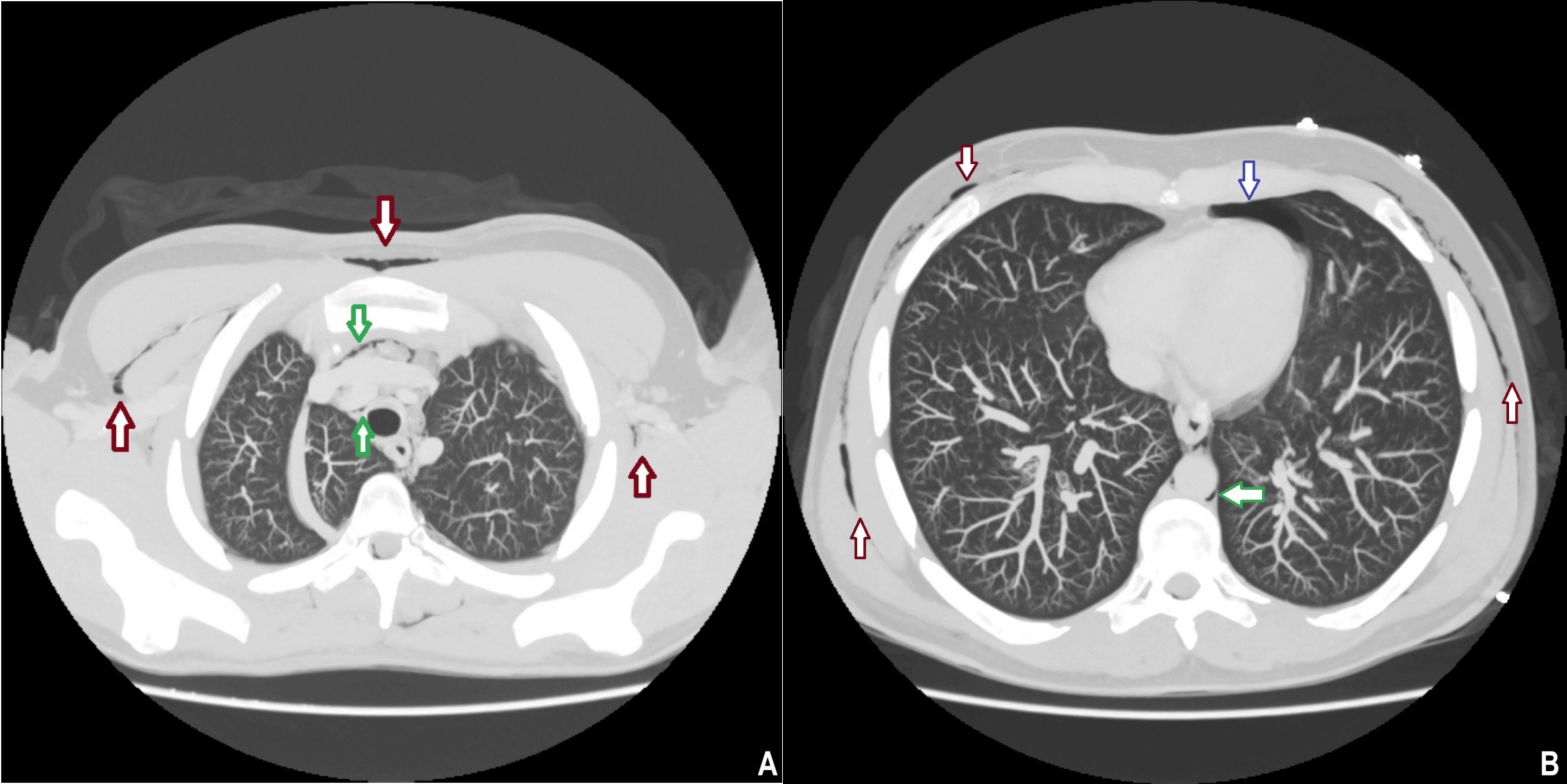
Axial CT of the thorax demonstrating extensive surgical emphysema (red arrows) in the chest wall, associated left-sided pneumothorax (blue arrow), and pneumomediastinum (green arrows) with air outlining the mediastinal structures. CT: computed tomography
